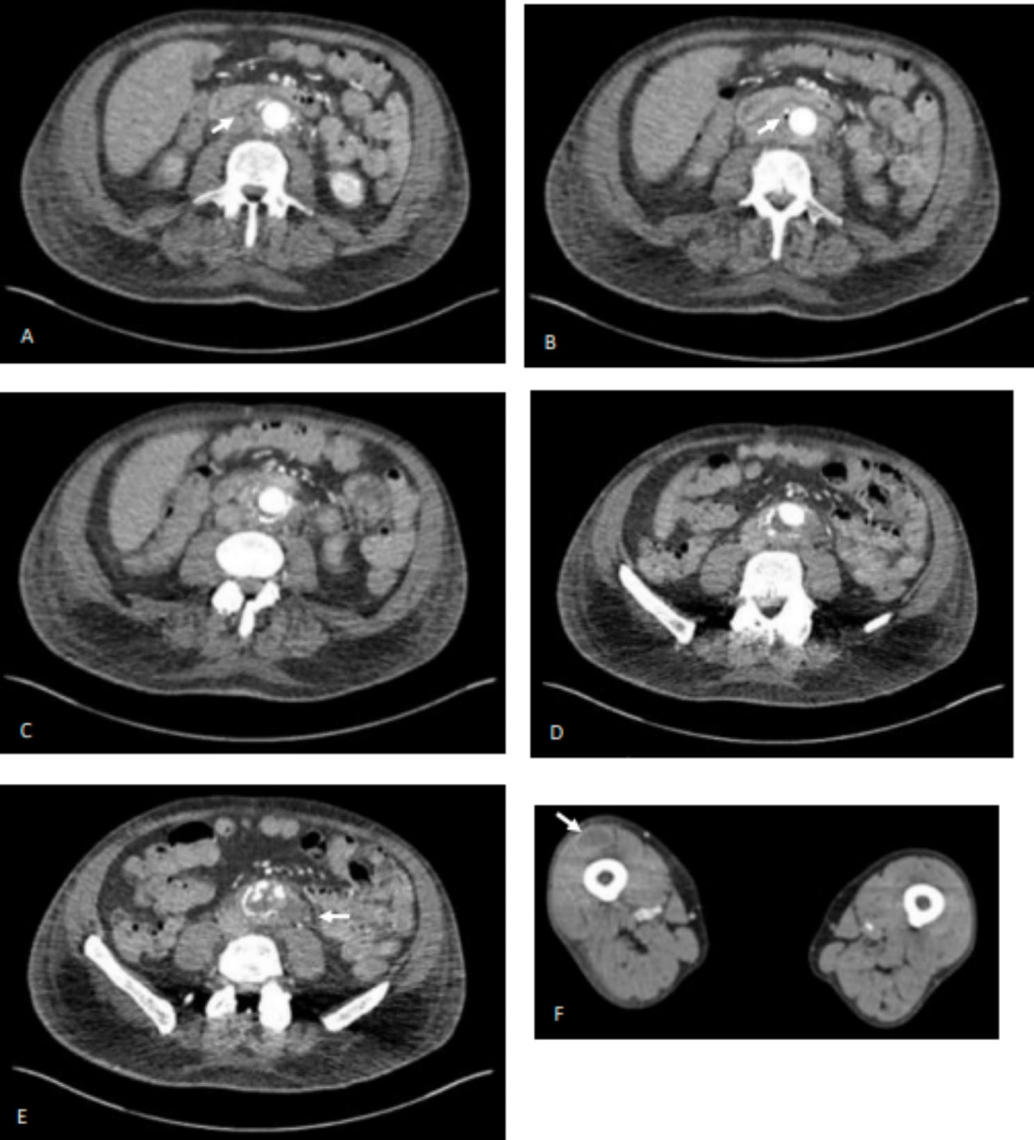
A-E represent caudal to rostal axial images from admission CT - aortic graft infection confirmed with locules of gas and multiple collections around the abdominal aorta. F shows right vastus lateralis collection
